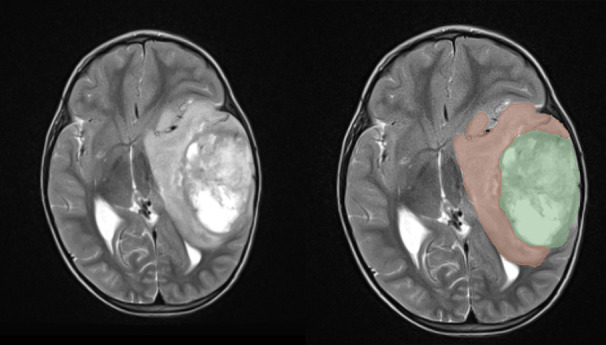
Contrast-enhanced MRI, T2 mode. Segmentation of the tumor of the left temporal lobe, the zone of the contrast-accumulating tumor core is highlighted in green, the zone of perifocal edema is highlighted in red. (Atypical teratoid rhabdoid tumor, GIV).
